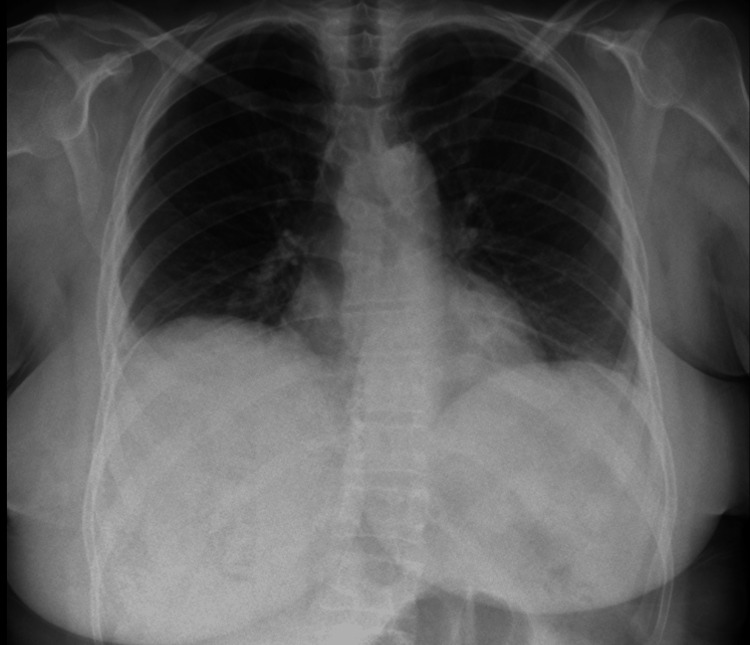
Chest X-ray with the resolution of the lung infectious process
